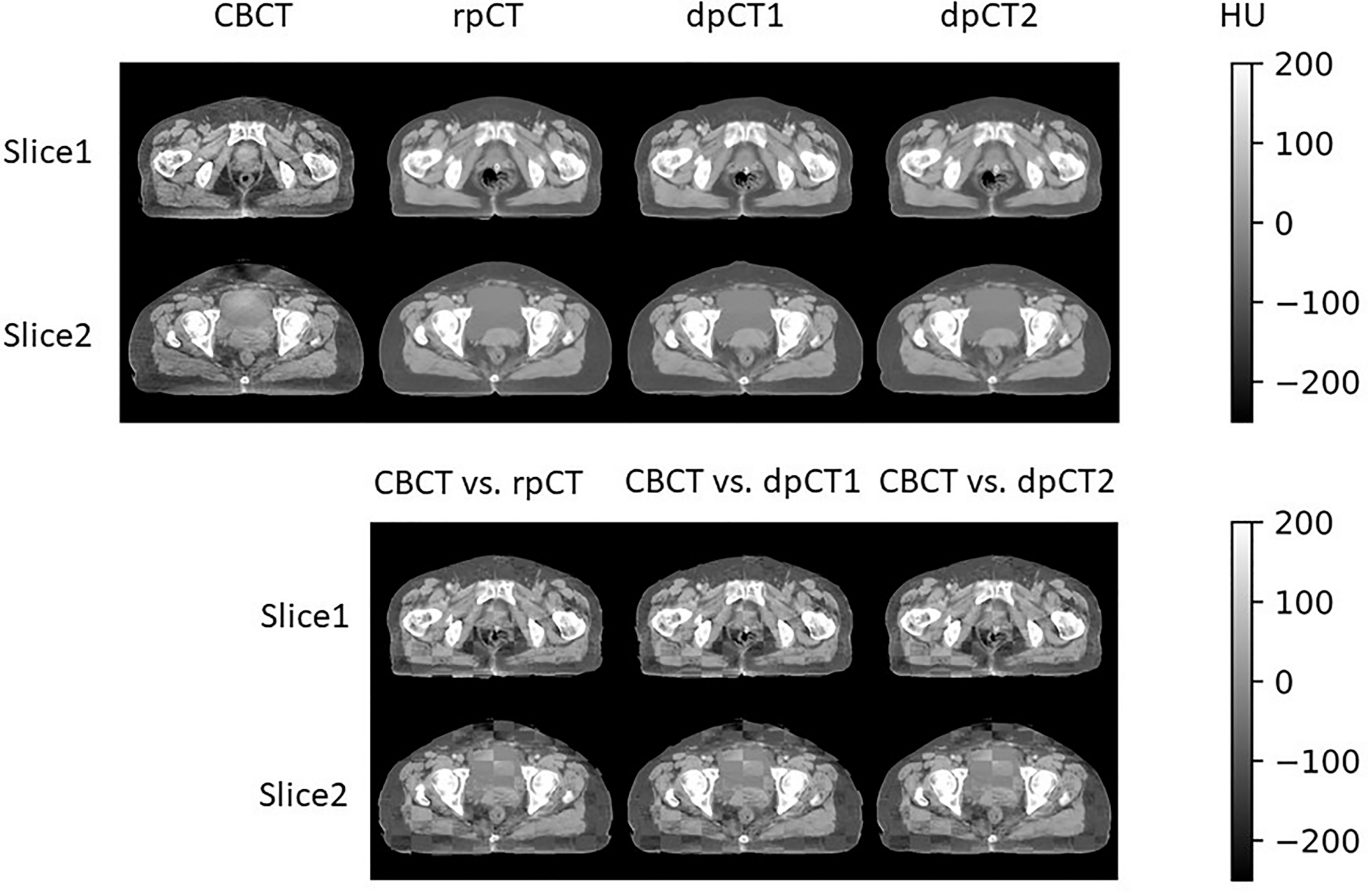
Visualization of registration result. rpCT, rigid planning CT; dpCT1, deformed planning CT with Elastix method; dpCT2, deformed planning CT with MSnet.
